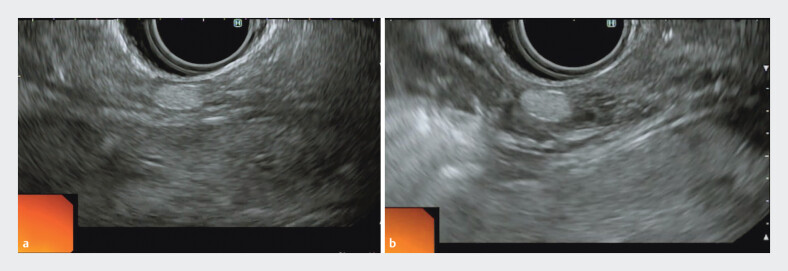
Endoscopic ultrasonography of a subepithelial lesion.
